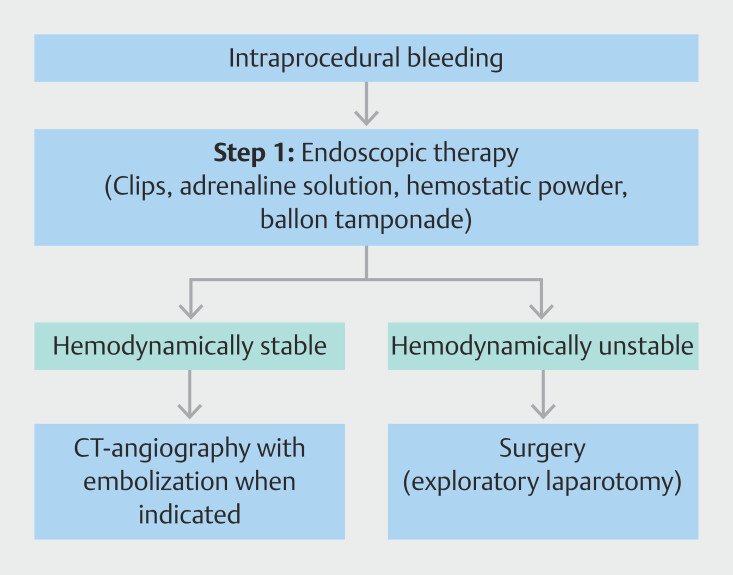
Management algorithm for intraprocedural bleeding during endoscopic necrosectomy. Stepwise approach including initial endoscopic hemostasis (adrenaline–saline solution, clips, hemostatic powder, balloon tamponade), followed by CT angiography with embolization if the patient is stable, and surgical exploration in unstable patients or if prior management fail.
